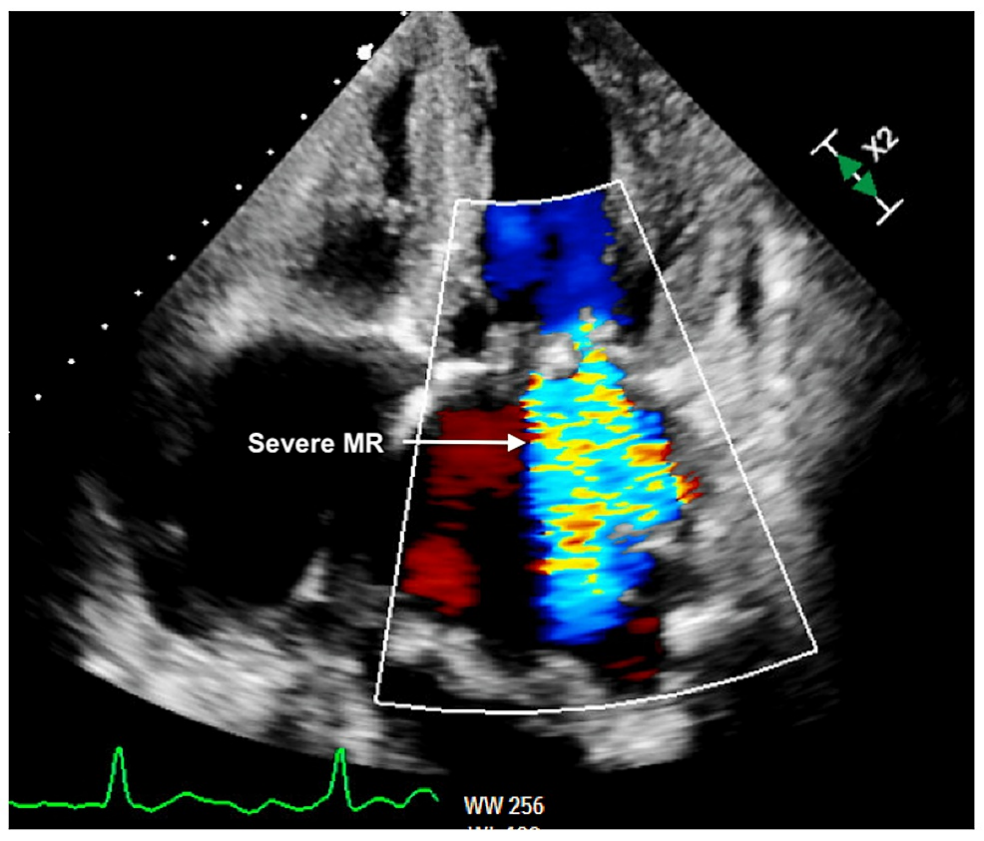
Transthoracic echocardiography four-chamber view showing severe MR MR: Mitral regurgitation
